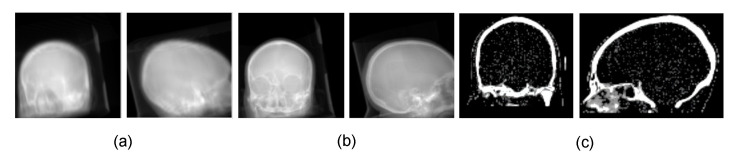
(a) DRRs of Case 3; (b) DRRs of Case 4; (c) CT image of Case 7. (a,c) are two failure cases using the proposed method. While (b) is a successful case.
